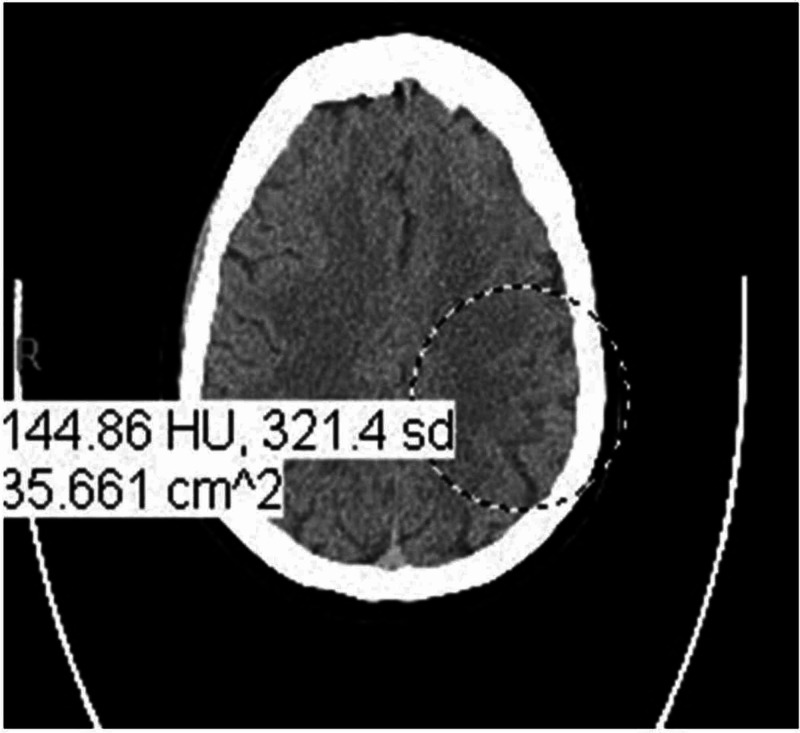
Non-contrast CT of the brain The image shows evidence of early left middle cerebral artery territory infarct CT: computed tomography
